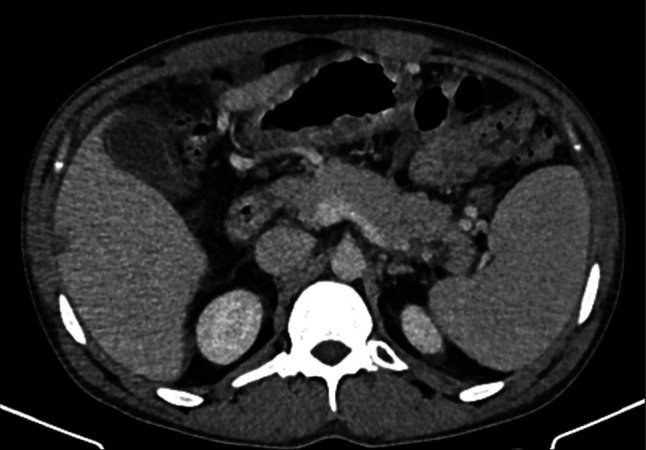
Follow-up CECT 2 years after anti-TB therapy, showing no residual lesions in the pancreas or ascites. CECT, contrasted enhanced computed tomography; TB, tuberculosis.
